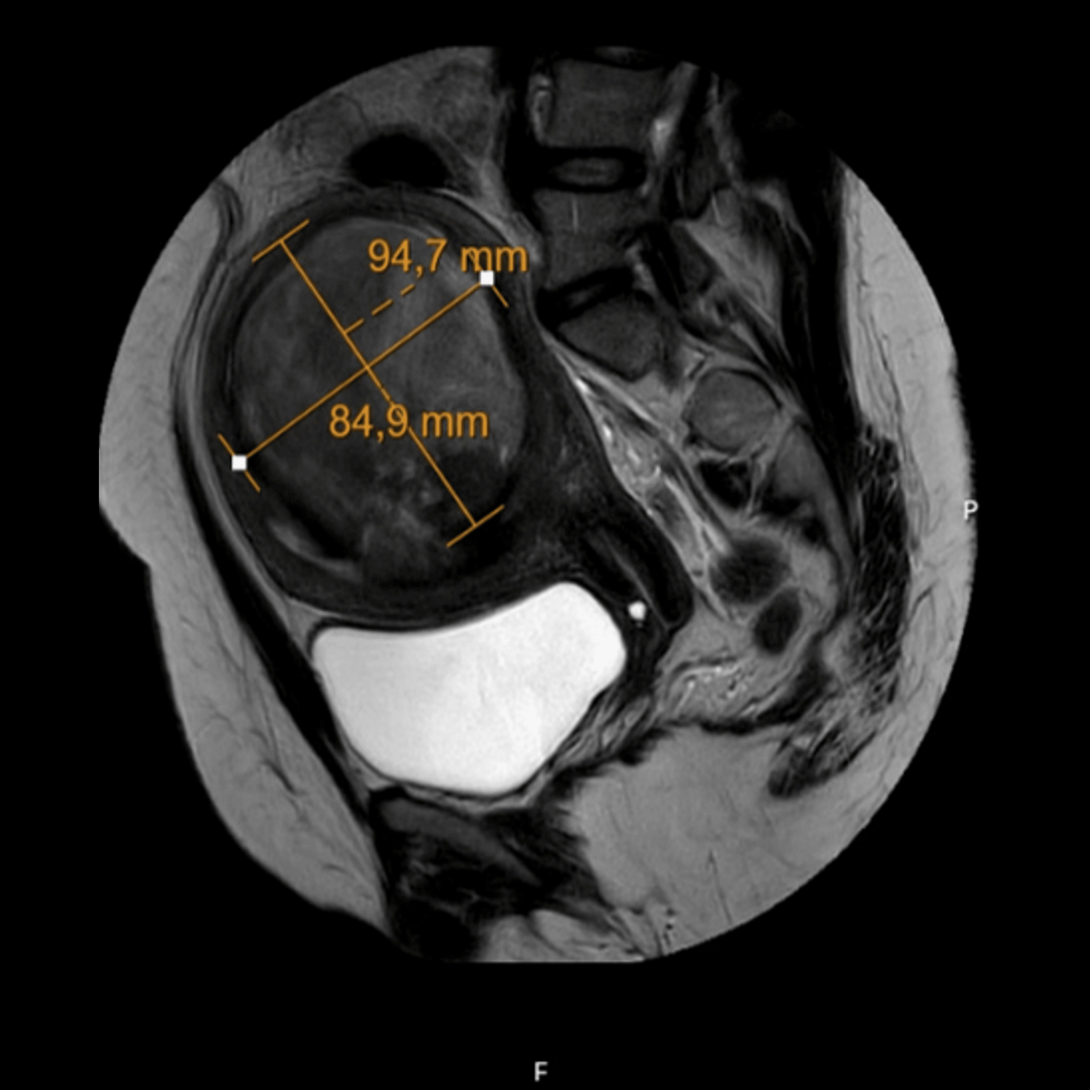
MRI six weeks after miscarriage
